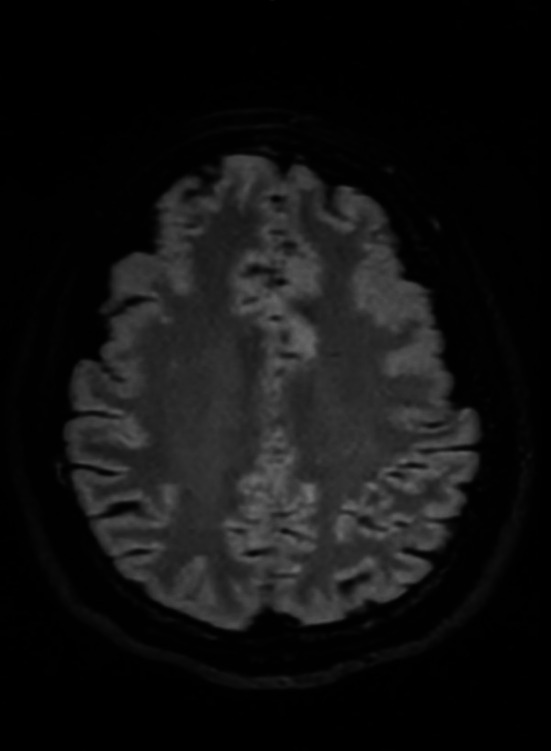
Axial brain MRI (T2‐FLAIR sequence) depicting bilateral frontal polymicrogyria, characterized by abnormal cortical thickening and irregular gyral patterns predominantly affecting the frontal lobes.
